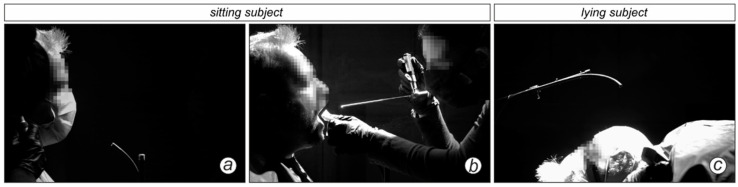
High-speed camera view on three exemplary tasks. (a) Otoscopy; (b) Rigid laryngoscopy through the mouth without a mask. (c) Sonography (supine) with MNC.
